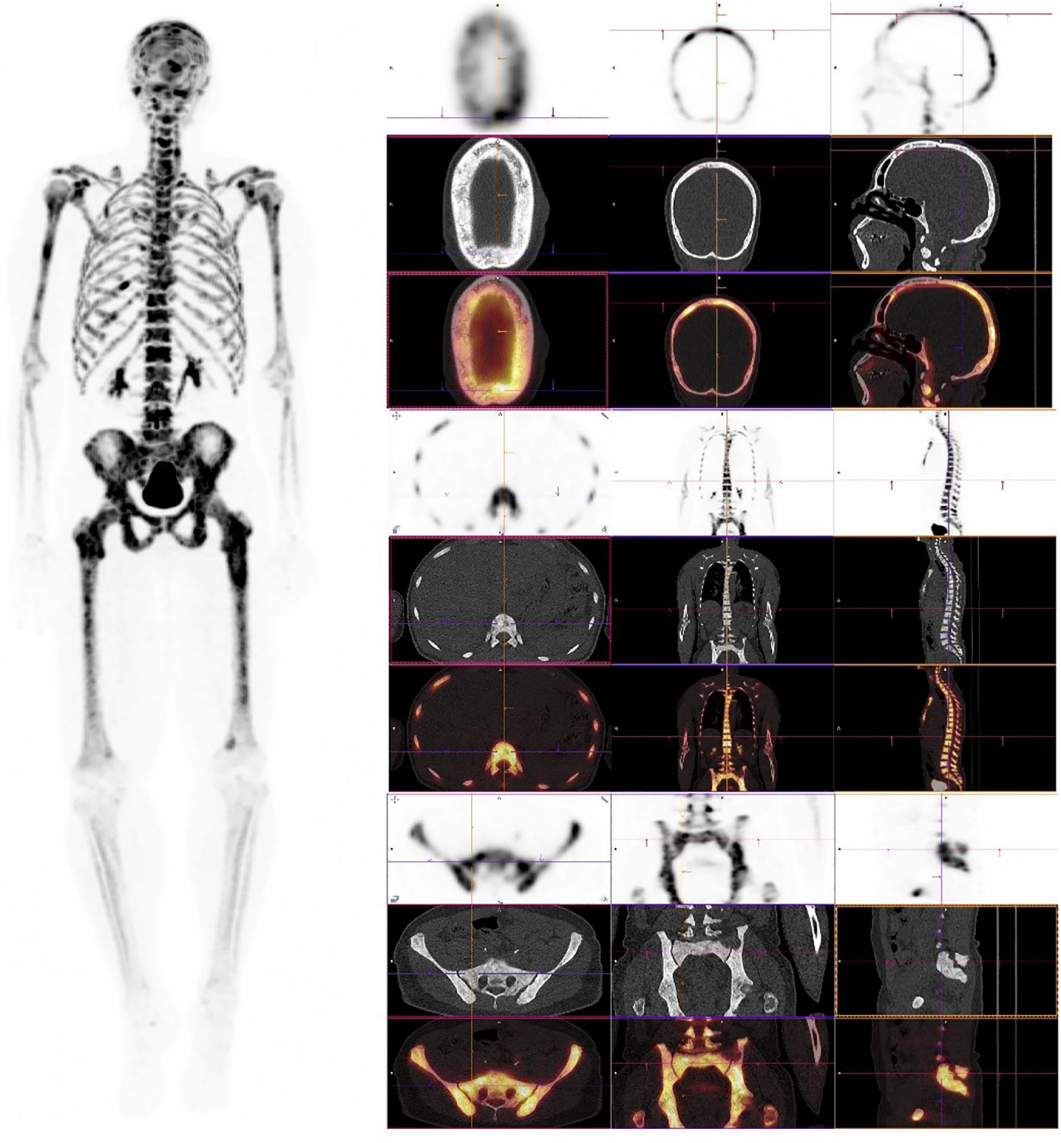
[18F]NaF PET/CT in secondary hyperparathyroidism with high-turnover metabolic bone disease. Left panel: Maximum intensity projection (MIP) shows diffusely increased skeletal uptake throughout the axial and appendicular skeleton with preserved renal visualization and prominent bladder activity, indicating normal tracer excretion rather than a classic superscan pattern. Right panels (low-dose CT and fused PET/CT): Cranial: Diffuse uptake in the calvarium and facial bones with a characteristic “salt-and-pepper” appearance on CT. Axial skeleton: Prominent uptake in vertebral bodies, ribs, and sternum, consistent with globally increased bone turnover. Appendicular skeleton: Increased uptake in the pelvis, femora, and other long bones, aligning with cortical-predominant involvement seen in renal osteodystrophy. The pattern reflects diffuse high-turnover bone disease associated with secondary hyperparathyroidism—generalized increased uptake on PET with diffuse sclerosis, skull “salt-and-pepper” changes, and cortical thickening on CT; preserved soft-tissue and renal visualization helps distinguish this from a true superscan presentation.
